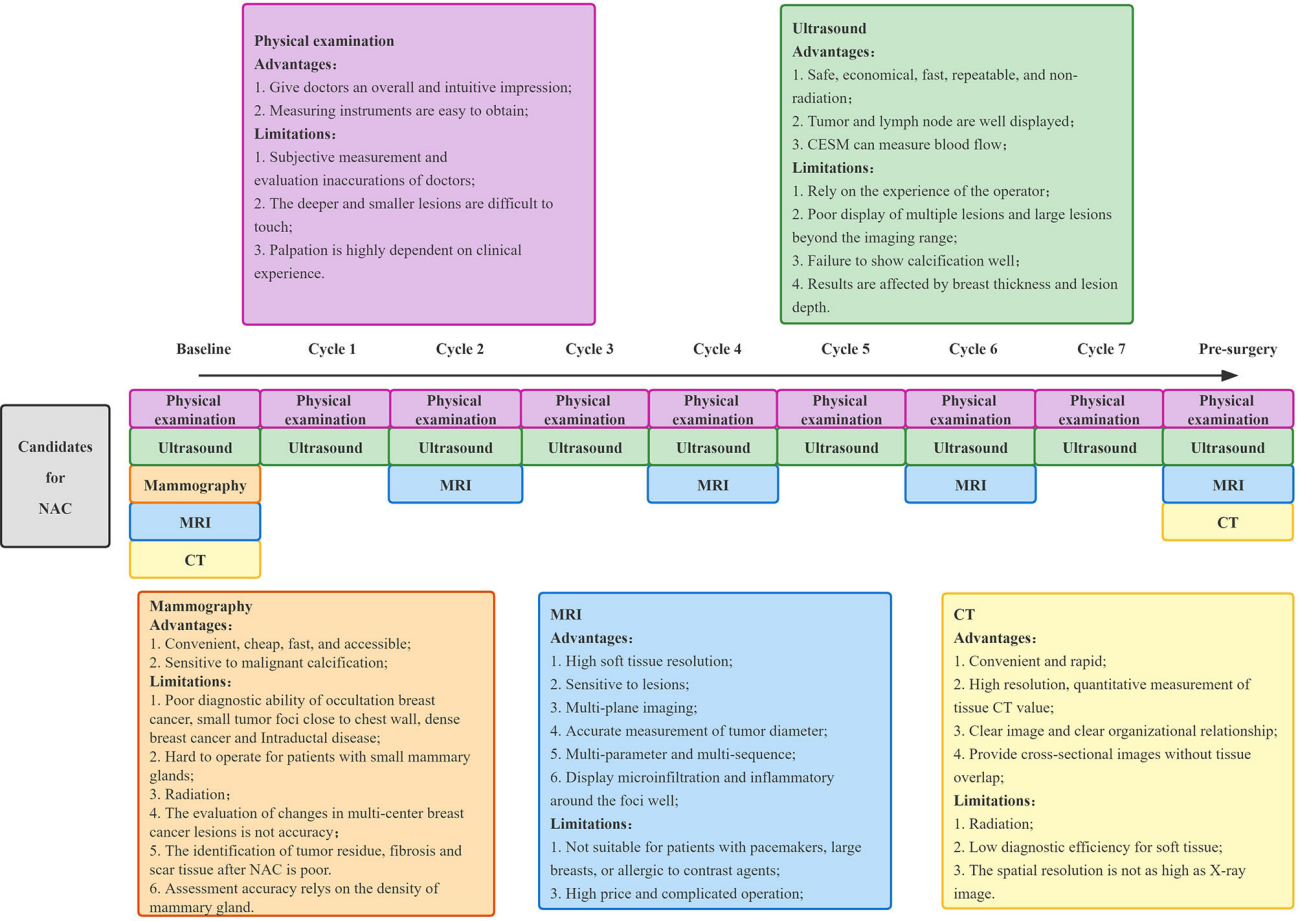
The model of imaging technology to assess the efficacy of NAC for breast cancer in Yunnan Cancer Hospital. NAC, neoadjuvant chemotherapy; MRI, magnetic resonance imaging; CT, computed tomography.
